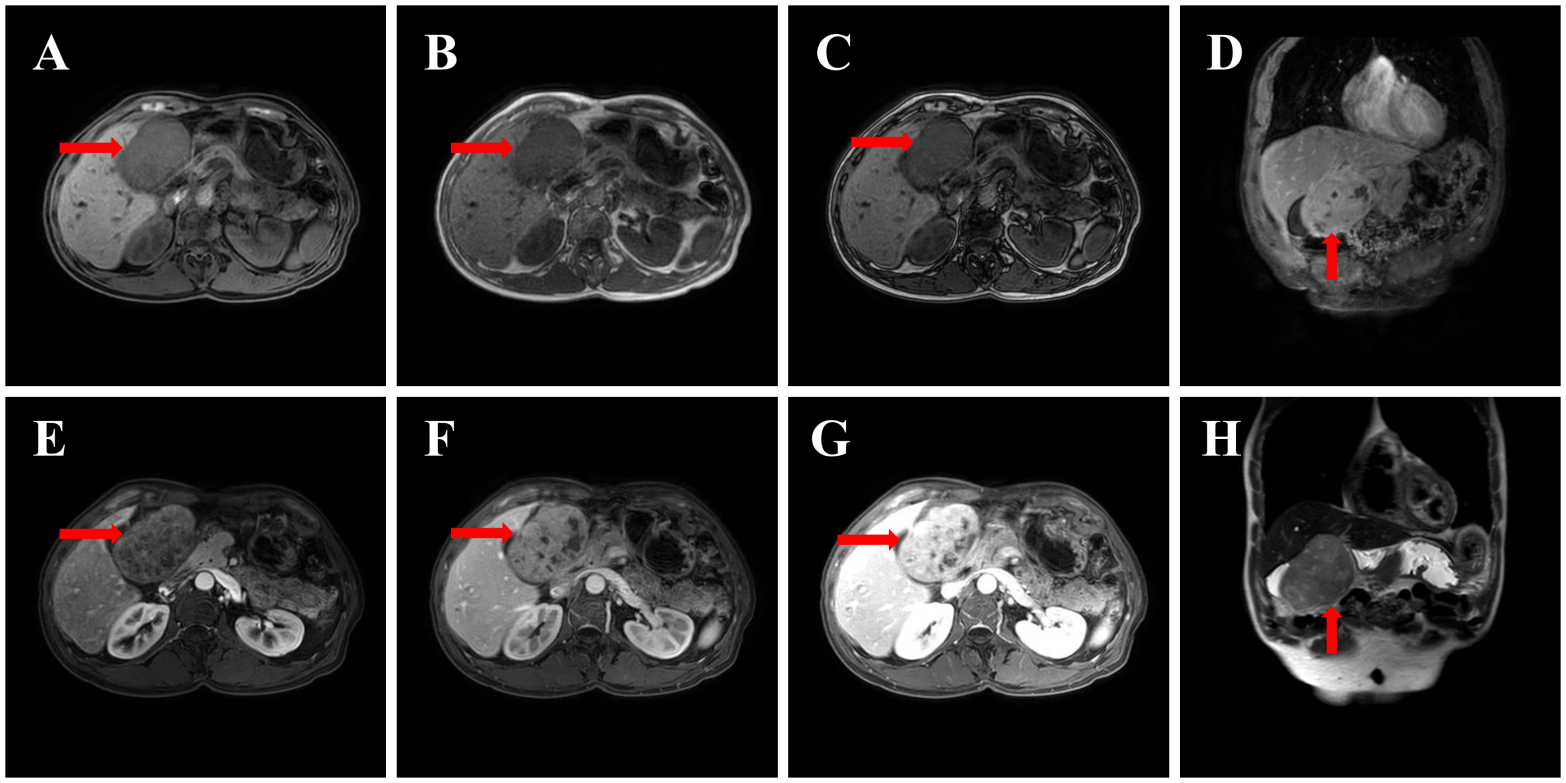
MRI image: (A) T1WI axial (mass isointense to liver, arrow); (B) T2WI axial (mixed signal, arrow); (C) DWI (restricted diffusion, arrow); (D) T2WI coronal (mass displacing gallbladder, arrow); (E) Contrast arterial phase (heterogeneous enhancement, arrow); (F) Contrast portal phase (enhancement progression, arrow); (G) Contrast delayed phase (capsular enhancement, arrow); (H) T1WI sagittal (mass-gallbladder relationship, arrow).
